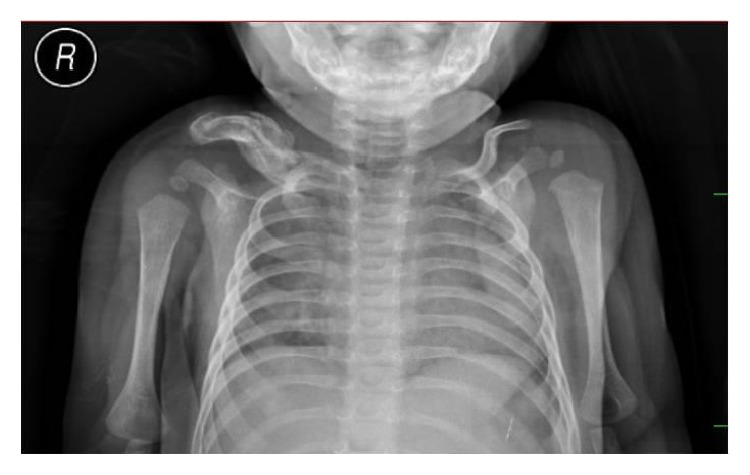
Radiograph showing extensive active osteomyelitis of middle third clavicle with sequestrum and involucrum.
